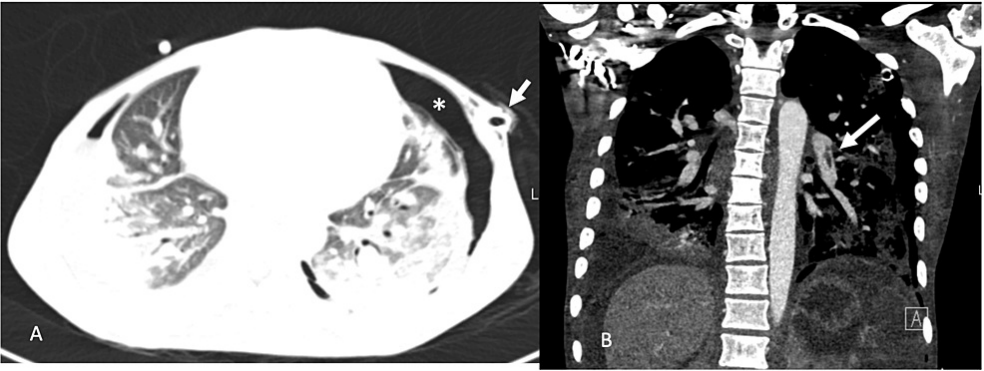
CT pulmonary angiogram of the patient at the time of presentation. (A) Bilateral pneumothoraxes indicated by the (*) on the left. Chest tubes were placed on presentation (white arrow points at the left-sided chest tube). (B) Central left-sided lobar pulmonary arterial filling defect indicated by the white arrow consistent with acute pulmonary embolism.
